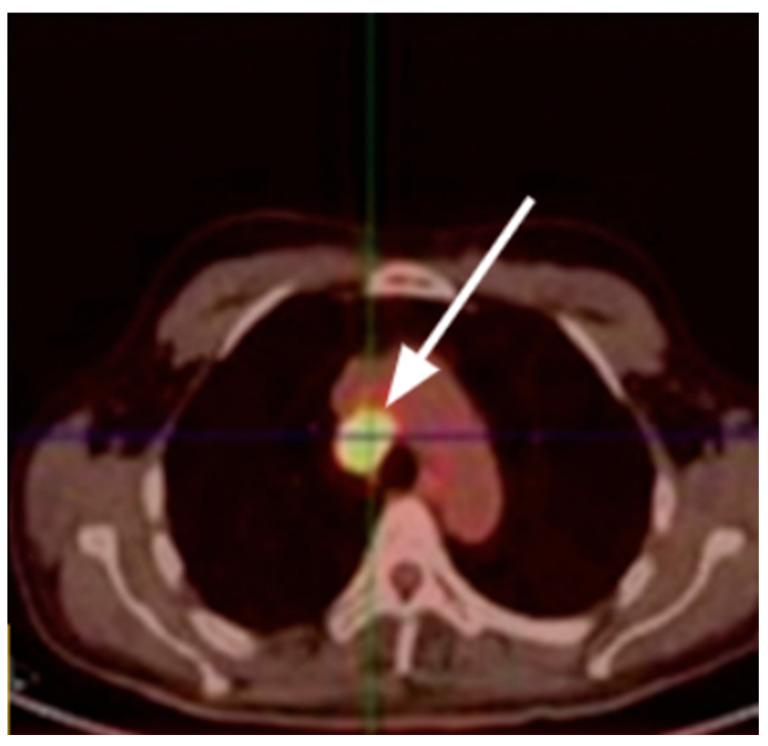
Axial PET/CT demonstrates hypermetabolic mediastinal lymphadenopathy (arrow) in LEMS. Clinical takeaway: Highlights PET/CT’s role in detecting thoracic malignancy—often small-cell lung cancer—as the underlying cause of LEMS, enabling early diagnosis and targeted management. Source [127].
